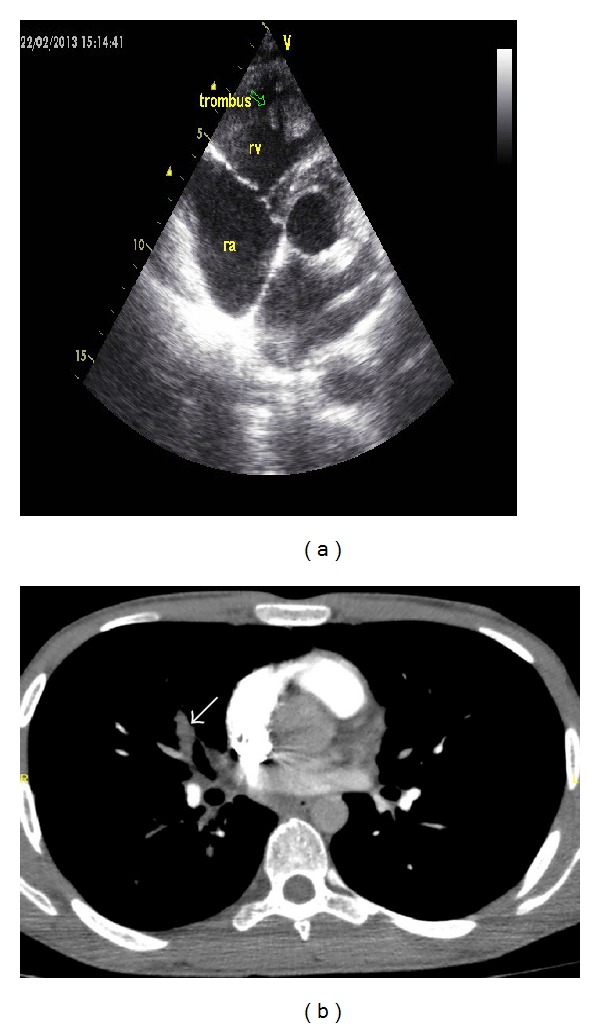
(a) Transthoracic echocardiography in the parasternal short-axis view shows a thrombus (arrow) in the right ventricle attached to the interventricular septum. (b) Contrast-enhanced pulmonary CT angiography shows acute embolus filling and mildly enlarging the right middle lobe artery (arrow).
